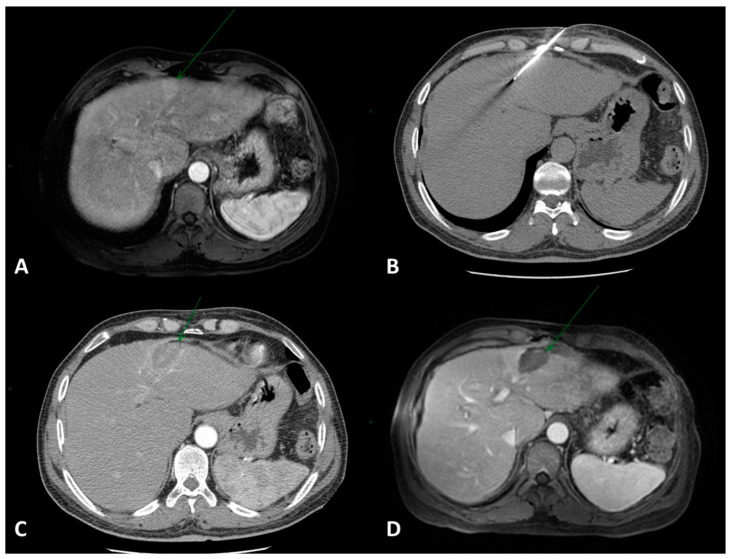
(A) Contrast-enhanced axial MRI showing a 2.5 × 2 cm HCC lesion in the IV hepatic segment (arrow). (B) CT-guided RFA was performed with one electrode. (C) Immediate post-procedural contrast-enhanced CT examination showing the ablation area without complications. (D) Post-treatment axial MRI showing complete response (arrow).
